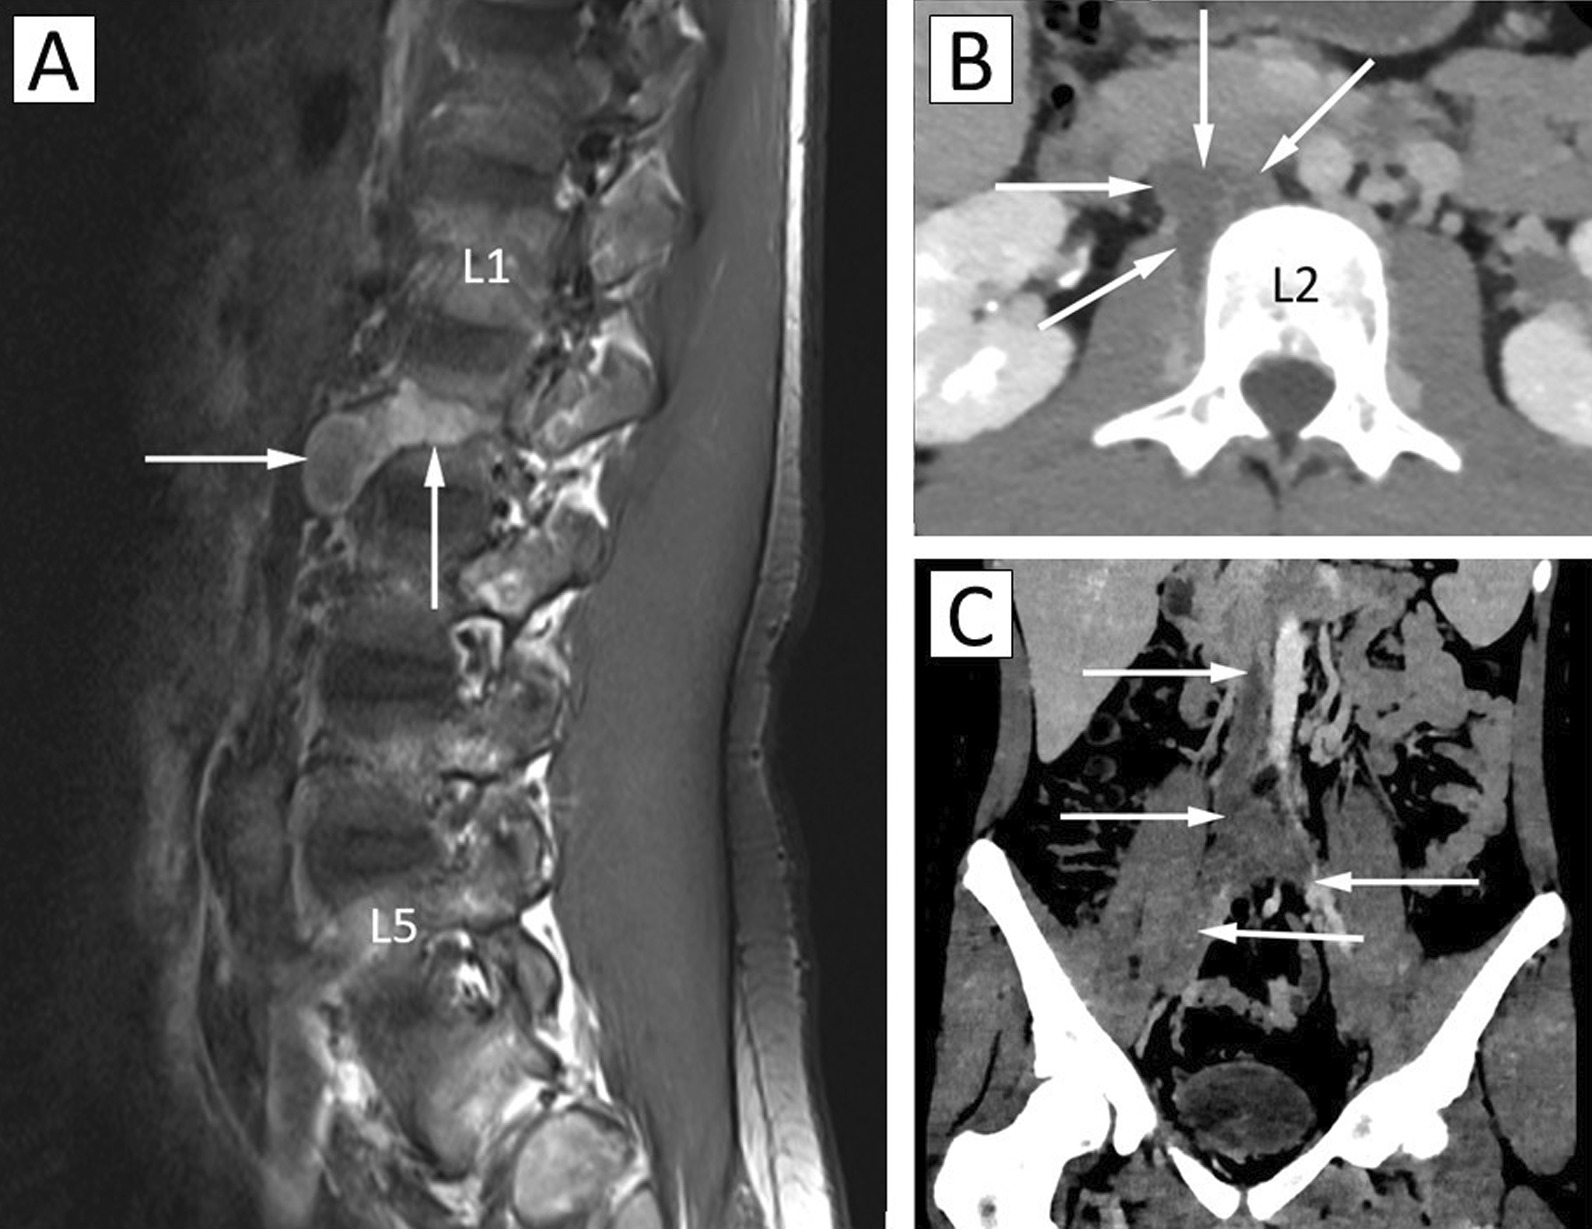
A Lumbar MRI (T1-weighted sagittal image). White arrows show the formation of a vascular venous abnormality adjacent to the L2 vertebral body in the laterovertebral right area, measuring 60 mm in its longest axis. B Abdominal CT (MonoE 50 keV—axial view). White arrows show a thrombus in the right L2 paravertebral vein. C Abdominal CT (with contrast—portal phase—MonoE 50 keV—coronal view). White arrows highlight the thrombosis of the inferior vena cava and two iliac veins
